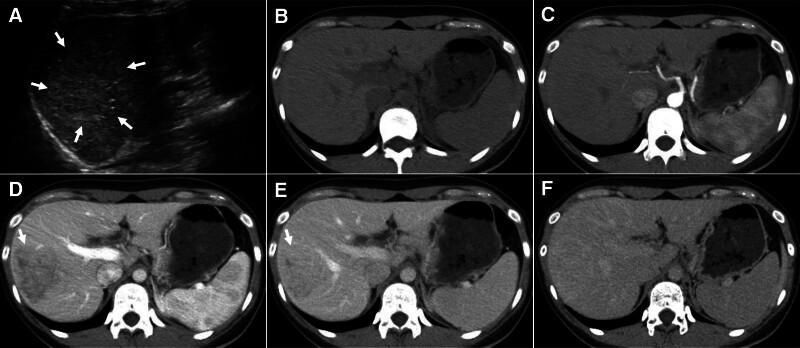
Ultrasonography and CT imaging of peliosis hepatis. (A) Ultrasonographic findings showing a slightly hyperechoic, ill-defined, round lesion in the right hepatic lobe (arrows). (B) Non-contrast CT showing no abnormal density within the liver. (C) Arterial phase demonstrating a patchy iso- to mildly hypodense lesion in segment VII of the right hepatic lobe. (D) Portal venous phase showing a heterogeneous hypodense lesion in segment VII (arrow). (E–F) Delayed phase with persistent centripetal enhancement, reduced lesion size, and ill-defined margins exhibiting isodensity (arrow).
